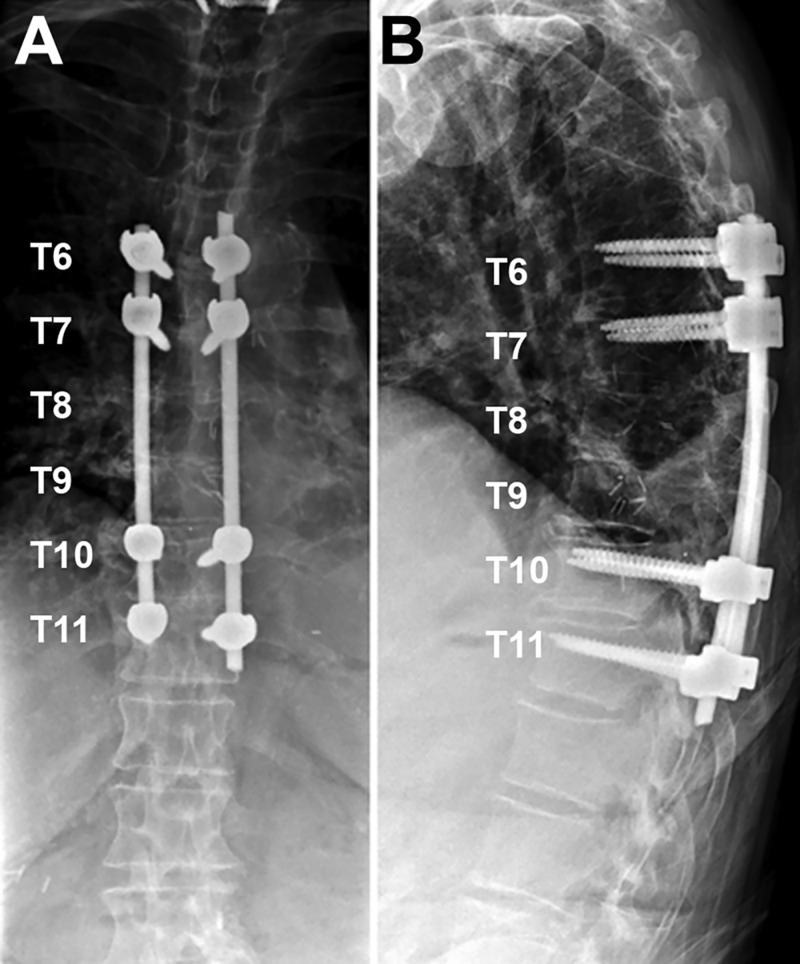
Postoperative X-rays demonstrating T6-11 posterior spinal fusion (A) anteroposterior X-ray. (B) lateral thoracic X-ray
